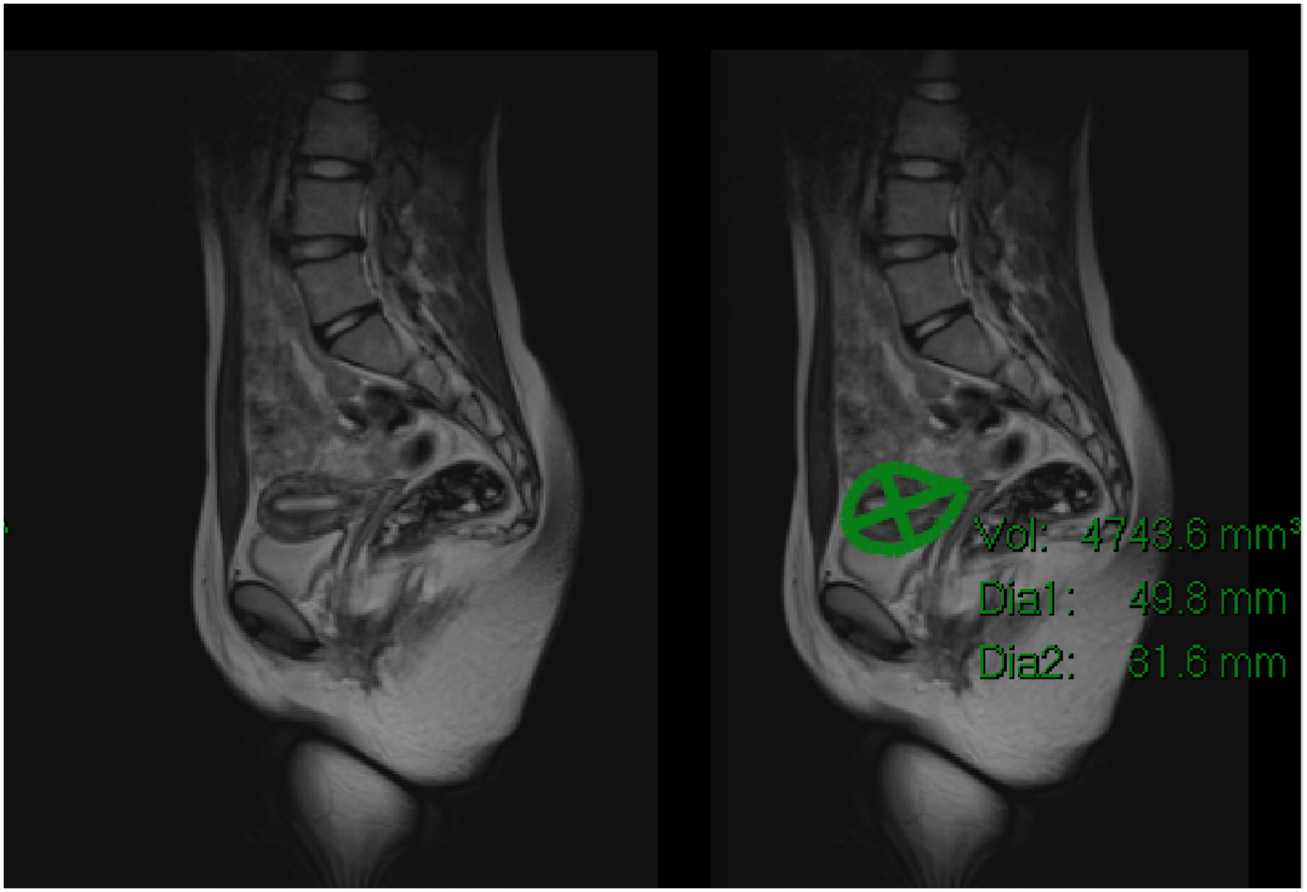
MRI pelvis with Sagittal T2 spin echo sequence demonstrating normal uterine morphology (left) with volume measurement (right) using a summation of area within the region of interest (ROI) from each contiguous image slice through the visualised uterus.
